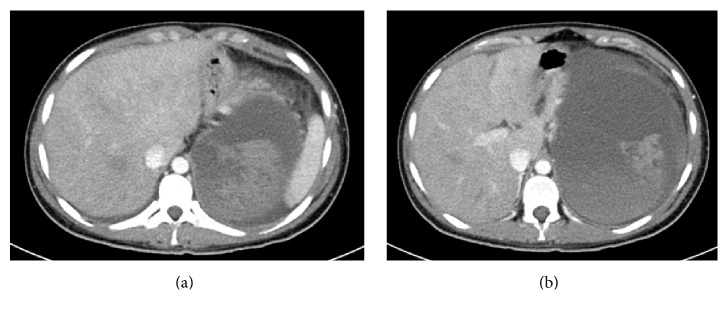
CT abdomen with contrast showing massive retroperitoneal haematoma of different ages.
